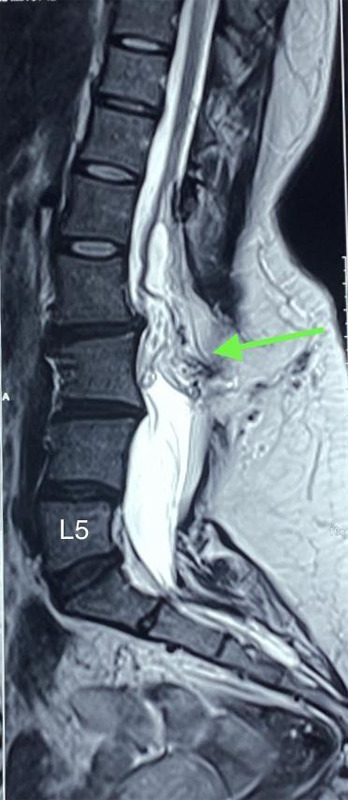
Contrast-enhanced MRI of the spine showing conus lying at the level of the lower end of L2 vertebra with herniation of epidural fat and small dural sac through defect in posterior elements at L3 level without herniation of neural components.
